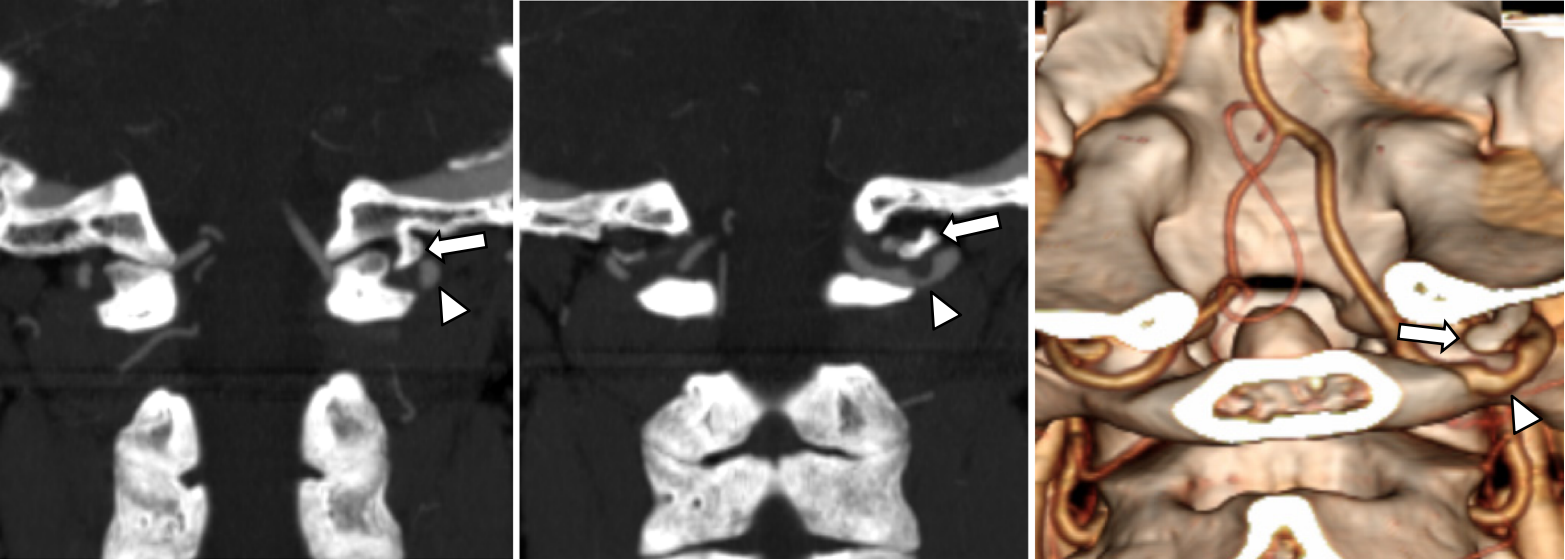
Anomalous occipital bony process compressing the vertebral artery. CT angiography showed the left vertebral artery (arrowheads) compressed by the anomalous occipital bony process (arrows).
